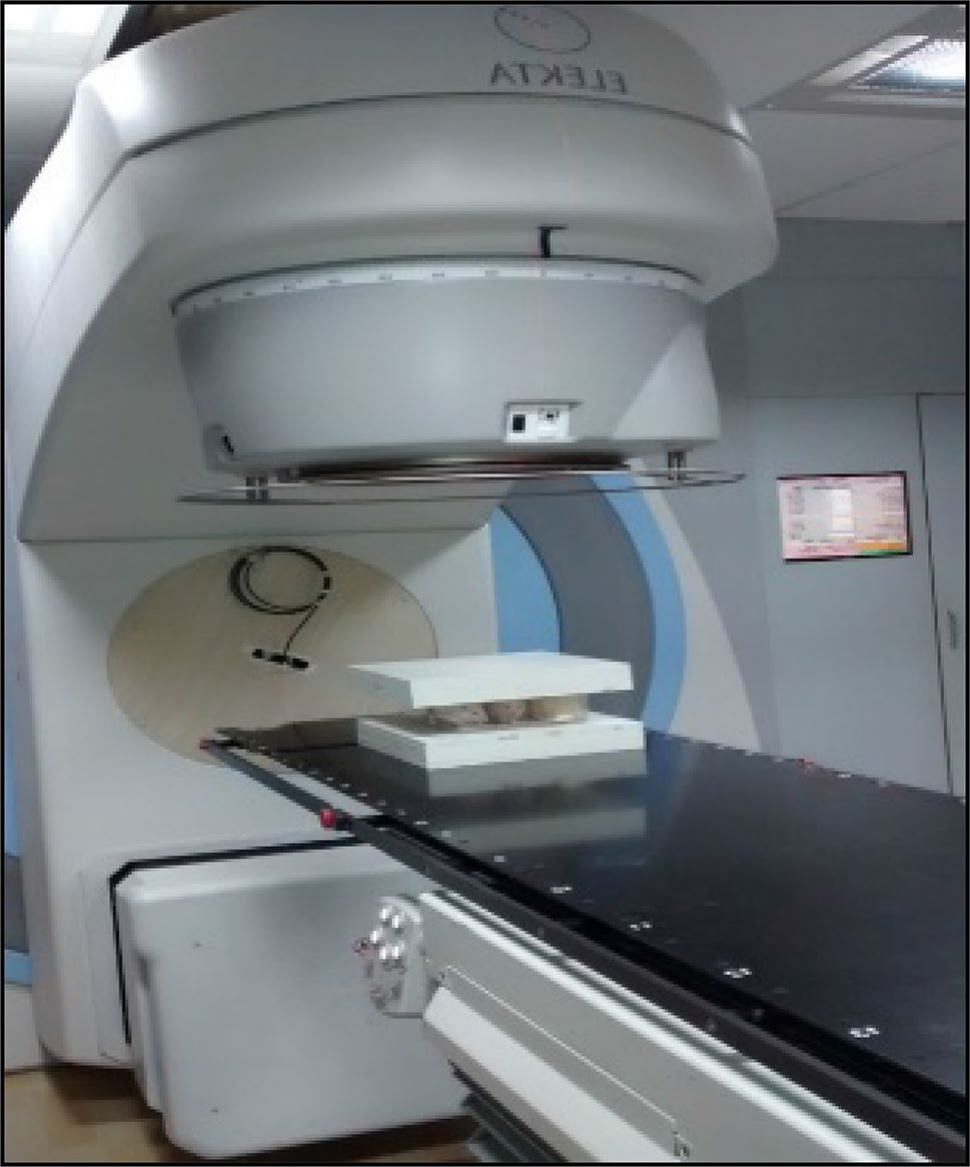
Procedural description of LINAC (Linear accelerator) whole-body X-ray exposure
